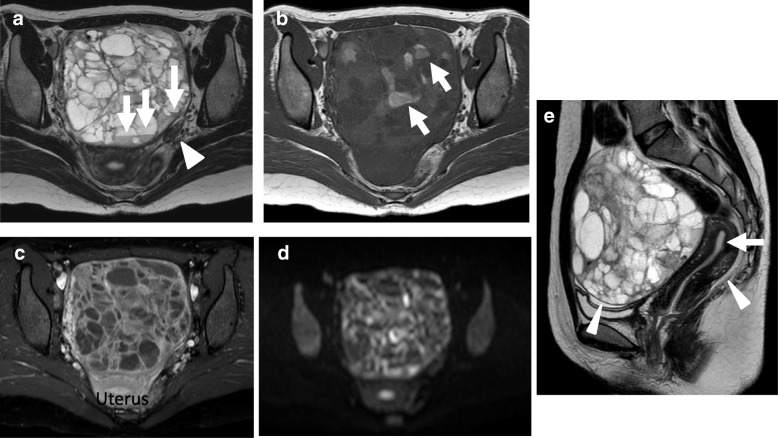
On MR images, a large, multiloculated right ovarian cystic mass, approximately 10 × 10 × 12 cm in size, was located at front of the uterus. a Axial T2-weighted image shows the multiloculated cystic lesion accompanied with thick septations. Fluid-fluid levels are demonstrated in several cystic components (arrows). The atrophic left ovary with few follicles is also detected (arrowhead). b Axial T1 weighted image shows intracystic high signal intensities suggesting intracystic hemorrhage (arrows). c On the contrast-enhanced axial fat-suppressed T1-weighted image, the septations demonstrated strong contrast enhancement similar to that of uterine myometrium. d Axial diffusion-weighted image demonstrates the septations having high signal intesnsities. e On sagittal T2 weighted image the uterus is normal size without endometrial thickening (arrow). There is a small amount of ascites which is limited to the pouch of Douglas and vesicouterine pouch (arrowheads)
